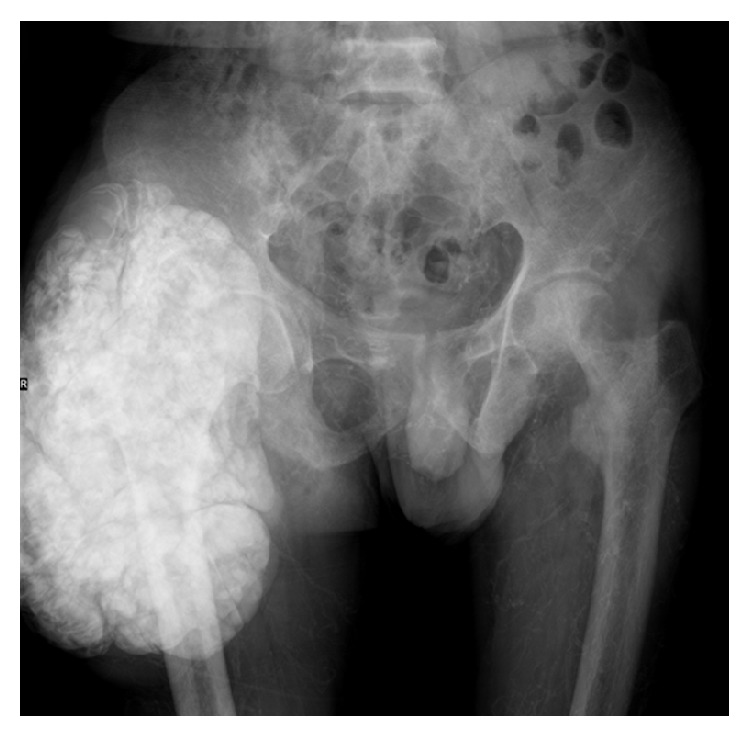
Pelvic X-ray indicated a high density mass, measuring about 26 × 18 cm in size near the right hip joint.
